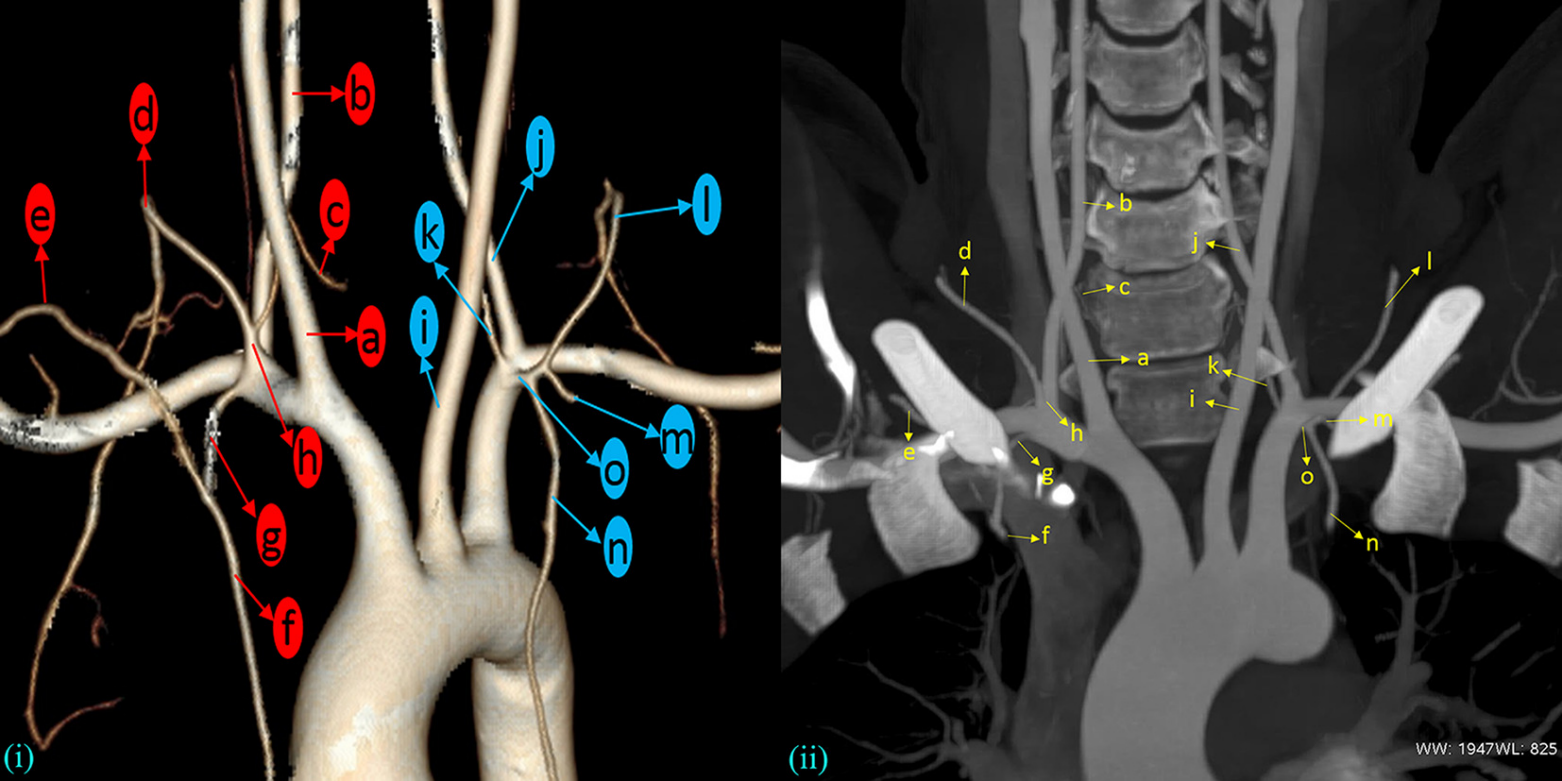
Three-dimensional reconstructed 64-slice CT angiogram (i) and coronal maximum intensity pixel reconstruction (ii) of the aortic arch and its branches. (a) Right carotid artery; (b) right vertebral artery; (c) right inferior thyroid artery; (d) right transverse cervical artery; (e) right suprascapular artery; (f) right internal thoracic artery; (g) common stump branching into (e) and (f); (h) thyrocervical trunk; (i) left carotid artery; (j) left vertebral artery; (k) left inferior thyroid artery; (l) left transverse cervical artery; (m) left suprascapular artery; (n) left internal thoracic artery; (o) common trunk branching into (l), (m) and (n).
